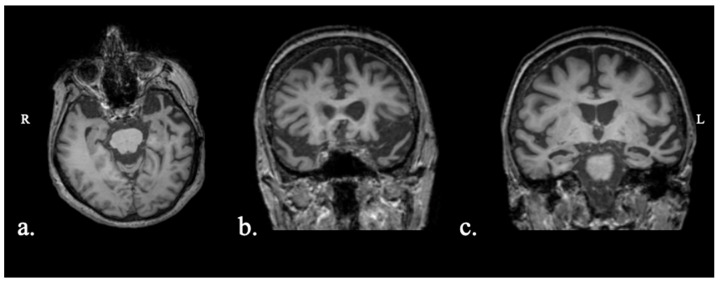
Patient ADY MRI scan: (a) axial view with bilateral temporal pole atrophy (L > R), with knife-edge appearance of left temporal pole gyri; (b) coronal view with pronounced bilateral temporal pole atrophy; (c) coronal view with moderate hippocampal atrophy bilaterally (Scheltens L: 3; Scheltens R: 2).
